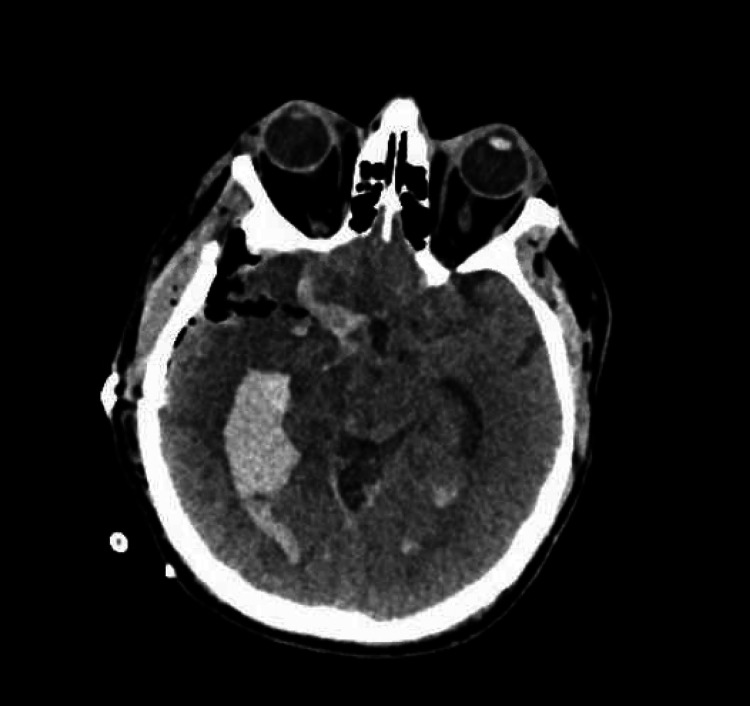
Postoperative noncontrast head computed tomography (CT) scan Postoperative CT scan of the surgical bed demonstrating hematoma evacuation and resection of the lesion.
